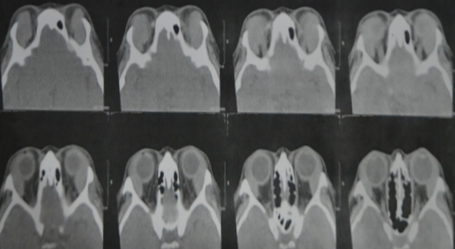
An axial view of a plain orbital CT scan of the patient showing a homogenous mass molding around the globe and recti bilaterally
